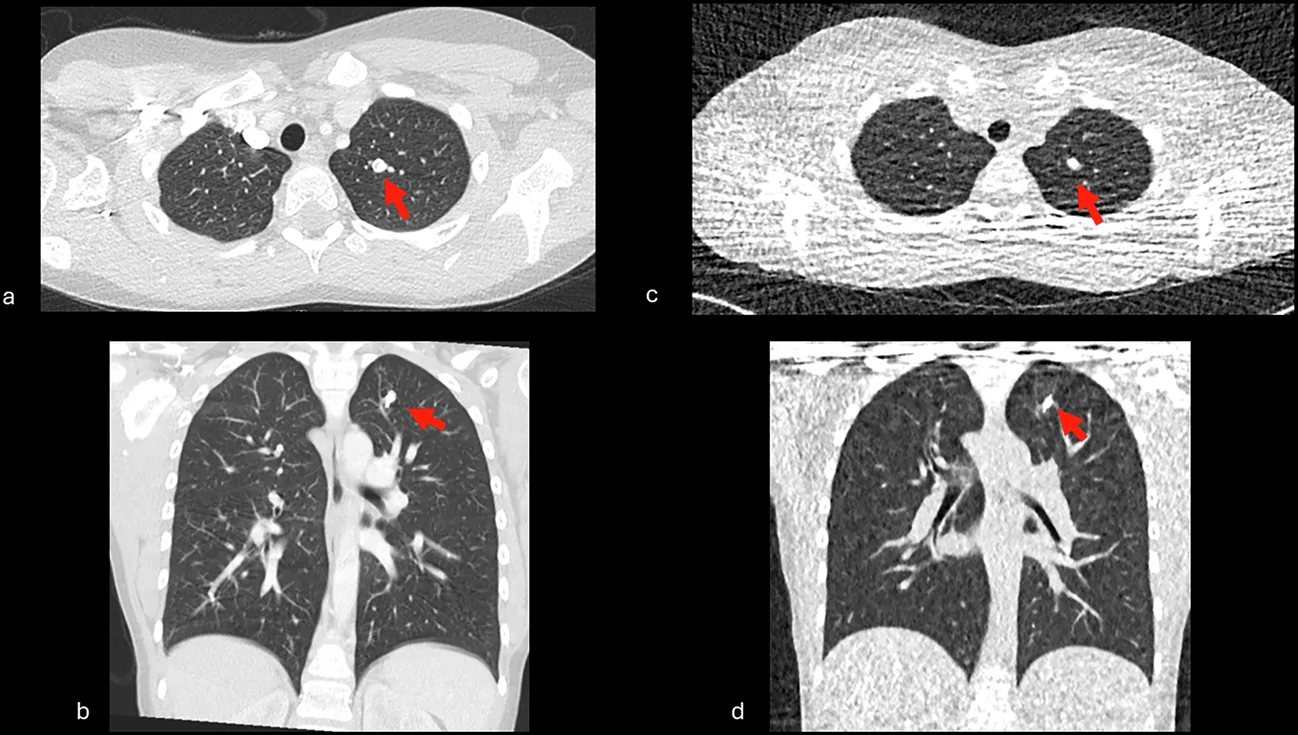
CT scans during follow-up of a patient known with a coiled pulmonary AV malformation in the left upper lobe (red arrow). Axial (a) and coronal (b) images obtained on energy-integrating-detector CT (with intravenous contrast) when the patient was 9 years old (CTDI 3.43 mGy, SSDE 6 mGy, WED 20.5 cm). Axial (c) and coronal (d) images obtained on PCCT using ULDCT protocol (without intravenous contrast) when the patient was 13 years old (CTDI 0.01 mGy, SSDE 0.02 mGy, WED 22.5 cm)
